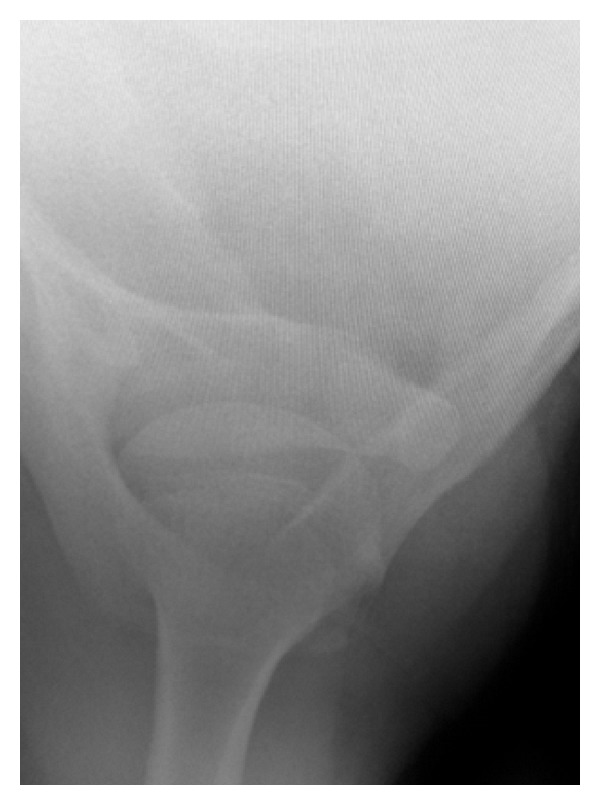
Case B: axillary radiograph at 12 weeks postinjury.
